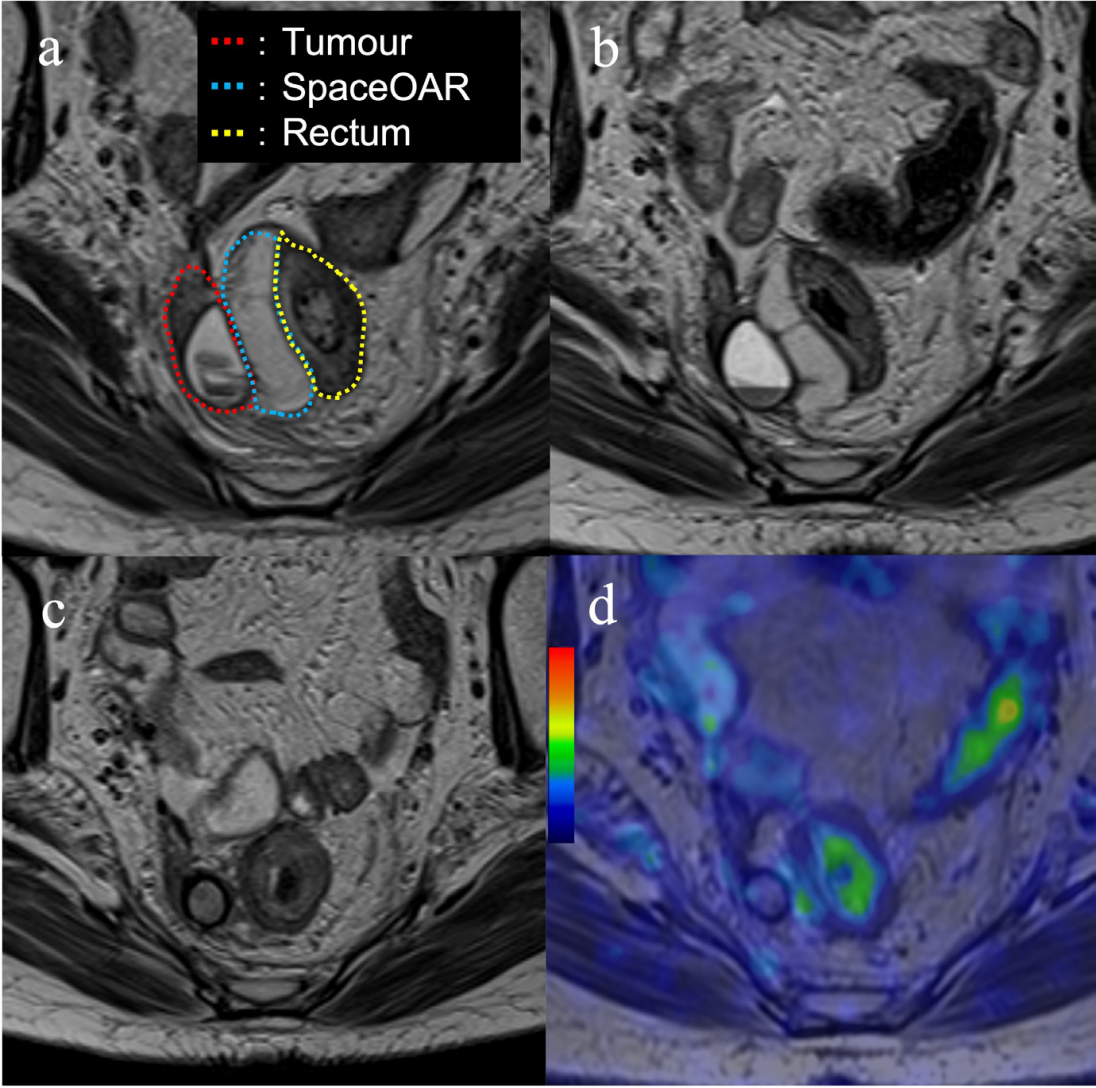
Images of the magnetic resonance imaging during and after treatment. (a) Four days after the first interstitial brachytherapy (red dotted line; tumour, blue dotted line; SpaceOAR, yellow dotted line; rectum); (b) One month after treatment; (c) Four months after treatment. The SpaceOAR is absorbed completely and has disappeared; (d) Image of the positron emission tomography-magnetic resonance imaging 8 months after treatment. There is no fluorodeoxyglucose accumulation in the recurrent tumour.
